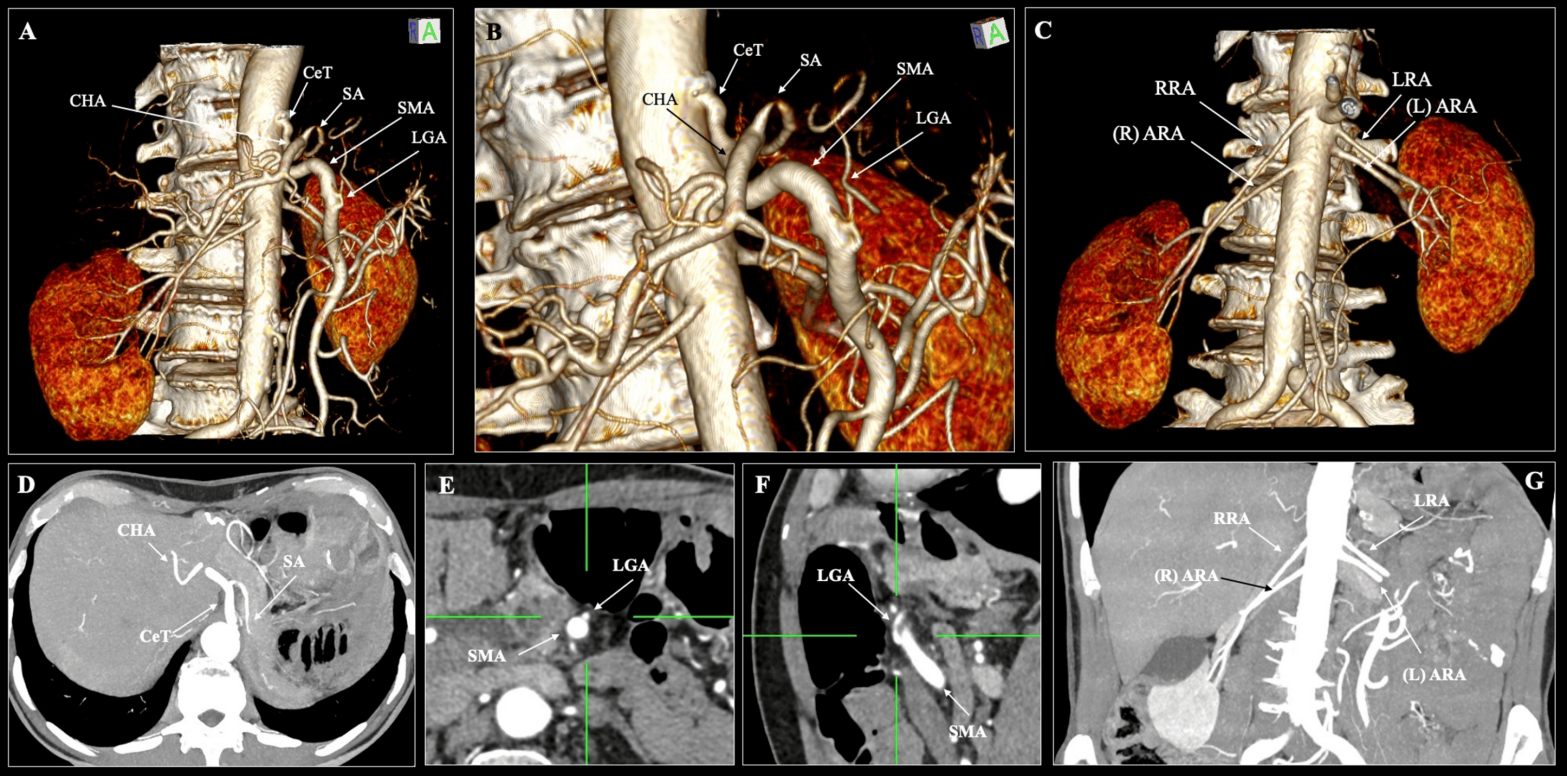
Computed tomography angiography (CTA) imaging of rare abdominal arterial variants. (A, B) Three-dimensional (3D) volume-rendered reconstructions demonstrate the co-occurrence of a hepatosplenic trunk (HST) and a gastromesenteric trunk (GMT). The coeliac trunk (CeT) bifurcates into the common hepatic artery (CHA) and splenic artery (SA). In contrast, the left gastric artery (LGA) arises anomalously from the superior mesenteric artery (SMA) forming a GMT. (C) 3D volume-rendered reconstructions demonstrate the bilateral accessory renal arteries (ARA). (D) Maximum intensity projection (MIP) of axial slice depicting the CeT bifurcating into the CHA and SA. (E, F) Multiplanar reconstruction (MPR) of axial and sagittal slices demonstrating the LGA origin from the SMA. (G) MIP of coronal slice depicting the bilateral ARA. CeT: coeliac trunk; CHA: common hepatic artery; SA: splenic artery; LGA: left gastric artery; SMA: superior mesenteric artery; RRA: right renal artery; LRA: left renal artery; ARA: accessory renal artery.
